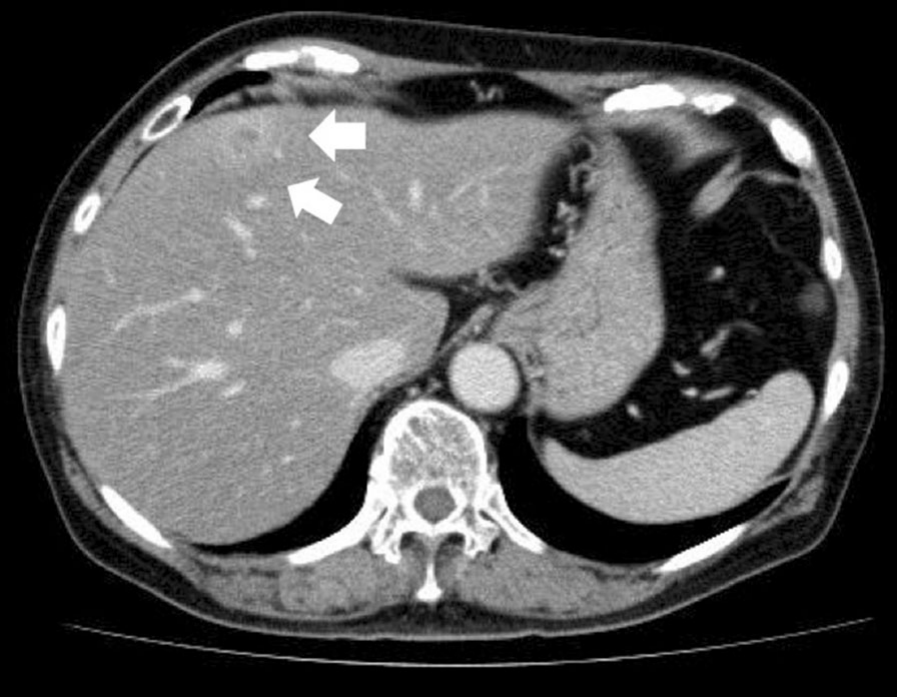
CT scan findings. CT scan revealed a 10-mm space-occupying lesion in the liver with ringed enhancement (*arrows*)
